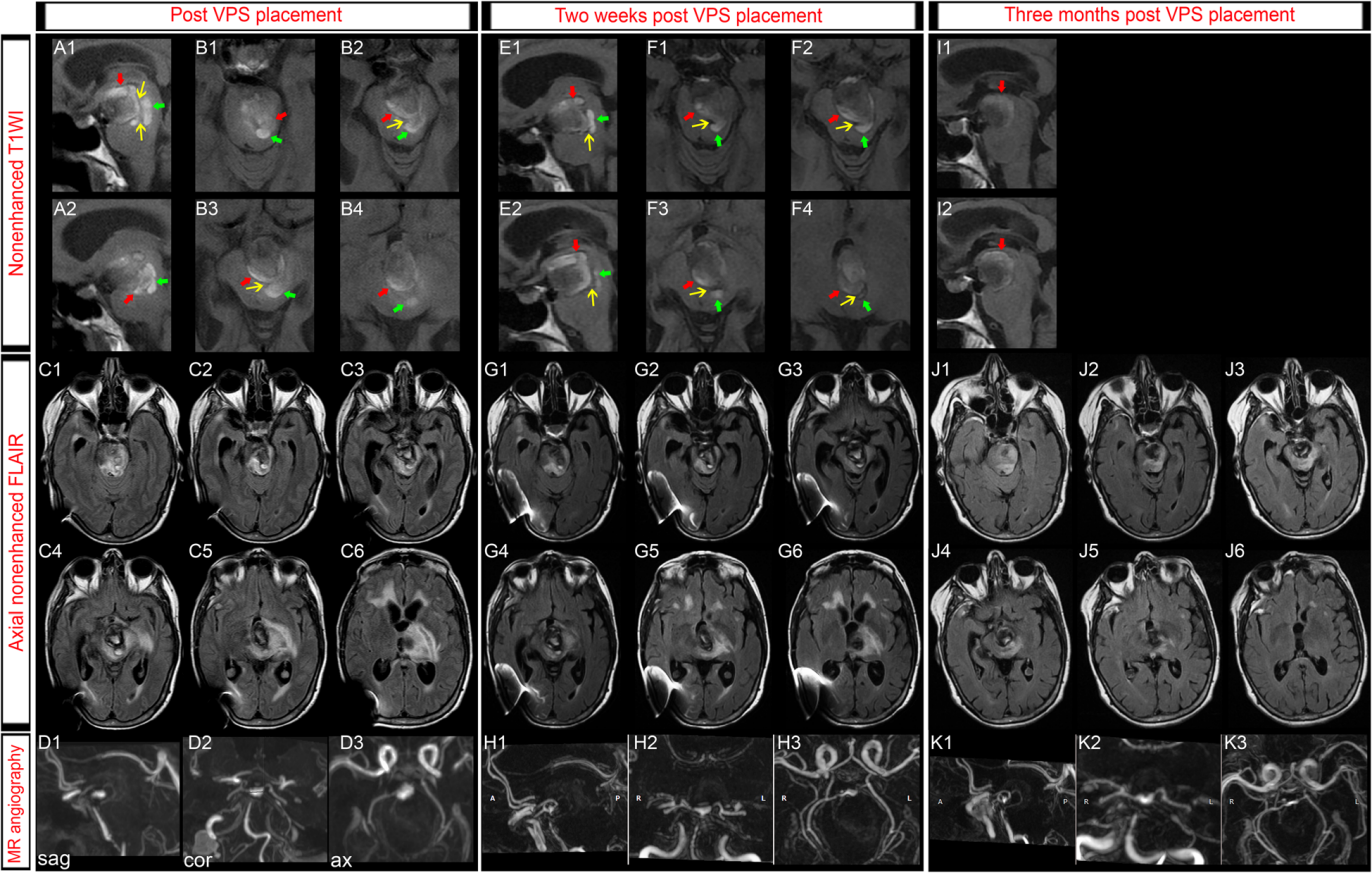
Magnetic resonance imaging (MRI) obtained on readmission to the hospital after temporary symptom relief. MRI demonstrated a large thrombosed aneurysm compressing the brainstem, with a hyperintense area indicating the presence of an acute clot within the aneurysm, besides markedly increased brain edema surrounding the enlarged aneurysm. a-b, e-f, i: Sagittal and axial nonenhanced T1-weighted images (T1WI) exhibited a high signal in the posterior portion of the aneurysm, suggestive of a fresh intraluminal thrombus (red arrows) as well as new intramural hematoma (green arrows; a-b), which caused a significant mass effect on the brainstem and third ventricle. The hyperintense areas on the images were located mainly on the contralateral side to the patent lumen. High signal on T1WI progressively decreased during follow up period (e-f, i), thus suggesting that no new hemorrhages happened. An intimal flap (yellow arrows in a-b, e-f) separated the true lumen and the false lumen. c, g, j: Axial fluid-attenuated inversion recovery (FLAIR) images showed a marked increase in brain edema in comparison with the previous MRI study. Intraluminal and intramural hematomas evolution were also detected on FLAIR images. d, h, k: Sagittal (sag), coronal (cor) and axial (ax) maximum intensity projection (MIP) reconstructions of magnetic resonance angiography (MRA) confirmed progressive thrombosis and reduced contrast filling in the residual blood lumen of the giant basilar apex aneurysm
